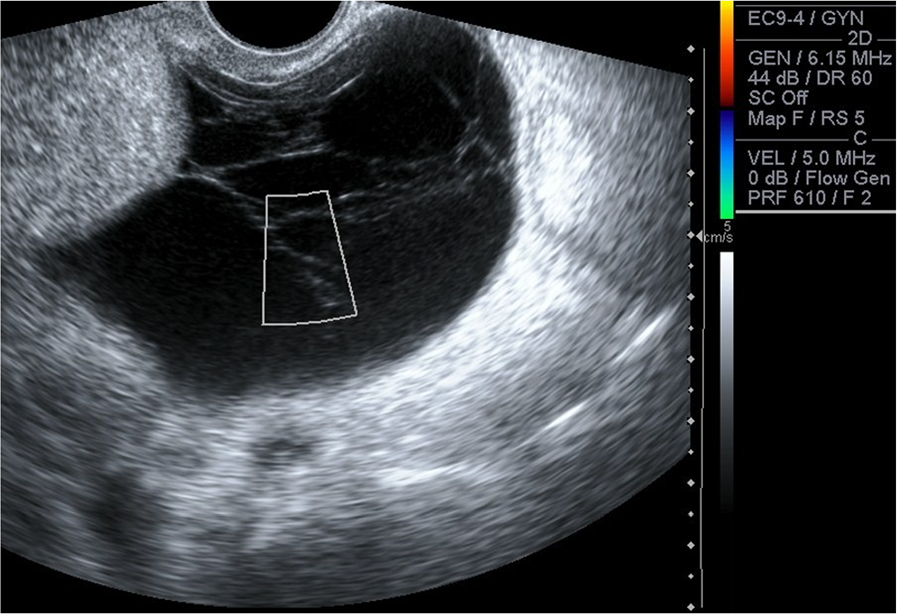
Transvaginal Ultrasound. Multilocular cystic left adnexal lesion measuring 7.6 cm x 7.0 cm x 6.1 cm. Septae are thin (<3 mm). No demonstrable solid or vascular component.
